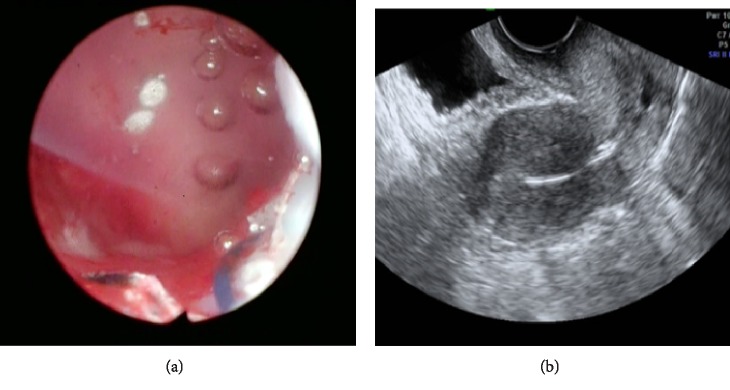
Hysteroscopic (a) and transabdominal ultrasound (b) findings for the intrauterine placement of the silicone plate.
